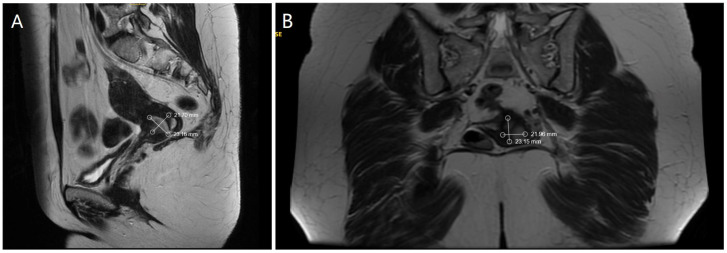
(A). MRI—Sagittal section of the tumor formation (B). MRI—Coronal section of the tumor formation.
